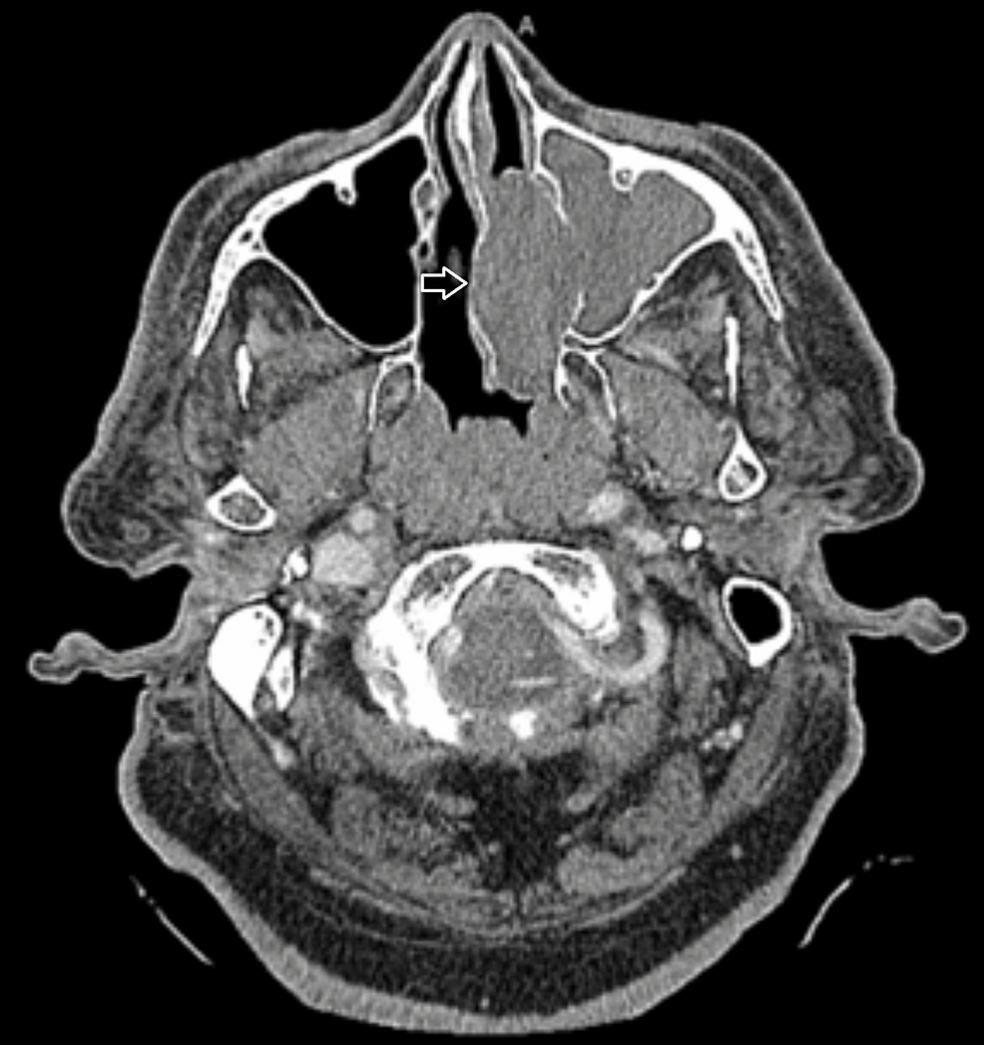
CT (axial view) of the paranasal sinuses showing non-enhancing soft tissue lesion in the left nasal cavity (arrow) extending to the left choana. The mass is causing mass effect and thinning of the nasal septum and medial wall of the left maxillary sinus. CT, computed tomography
